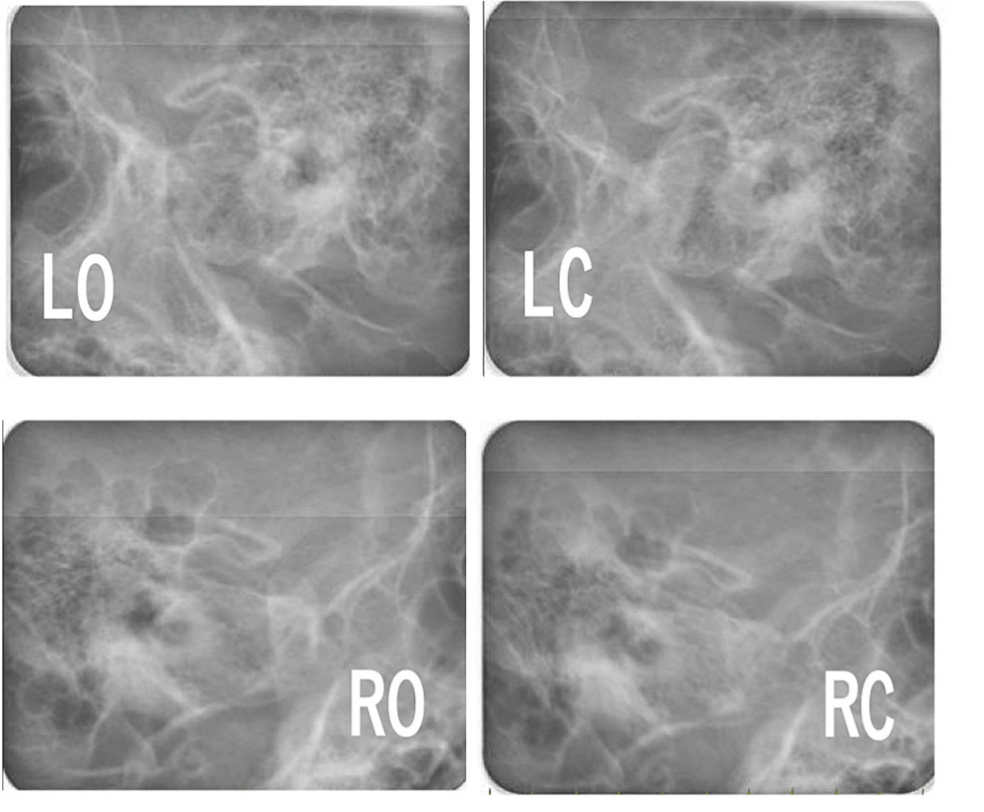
Presurgical evaluation. Schuller’s radiographs. LO, left open; LC, left close; RO, right open; RC, right close.
